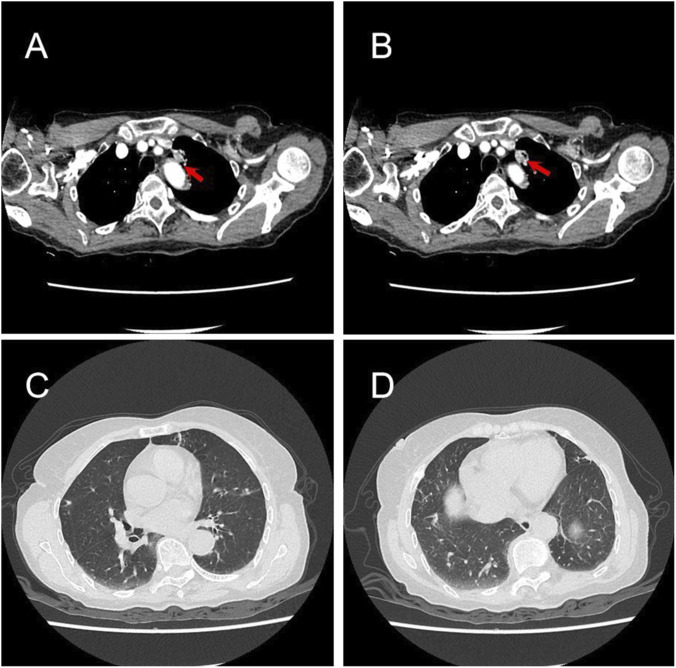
Radiographic response following 10 weeks of neoadjuvant almonertinib. (A,B) Post-treatment chest CT images reveal marked regression of the primary tumor (from 29 mm to 16 mm). Note the restoration of a clear fat plane between the lesion and the mediastinum (arrow). (C,D) Complete radiological resolution of COVID-19-related pulmonary infiltrates.
